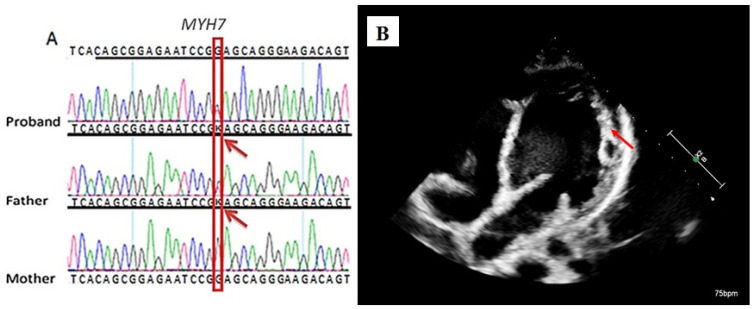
Familial nature of the cardiomyopathy in the studied case. Sanger sequencing validates the presence of chr14:23431858C>A, the *c.542G>T* variant of *MYH7* gene, in the proband and his father. K represents either G or T; nucleotide variants are indicated by the red arrow (A). Transthoracic echocardiography of the patient’s father at age 42. The red arrow is pointing at noncompact myocardium is visualized in the region of the apical and middle segments of the left ventricle (B).
